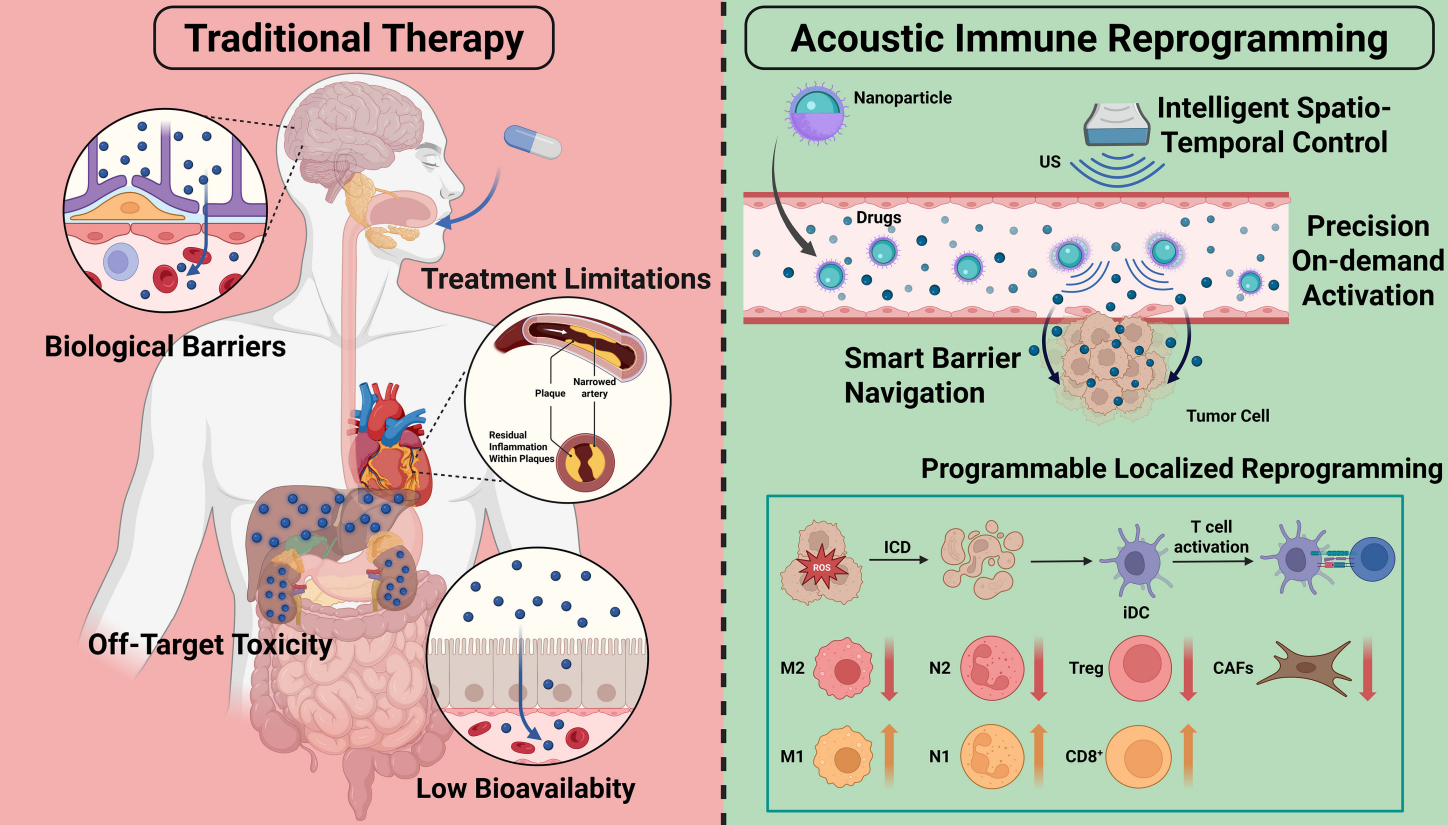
Definition of paradigm: the central concept of acoustic immune remodeling (Created in https://BioRender.com): Traditional therapies encounter significant challenges, including off-target toxicity (8), biological barriers (14), treatment limitations (13), and low bioavailability (15). Acoustic immune remodeling seeks to employ ultrasound to induce ICD through thermal and mechanical effects, or to modulate immune status by enhancing the permeability of drugs and cells (22). This approach offers several advantages, including “spatio-temporal control”, “on-demand activation”, “barrier breach”, and “localized reprogramming”.
